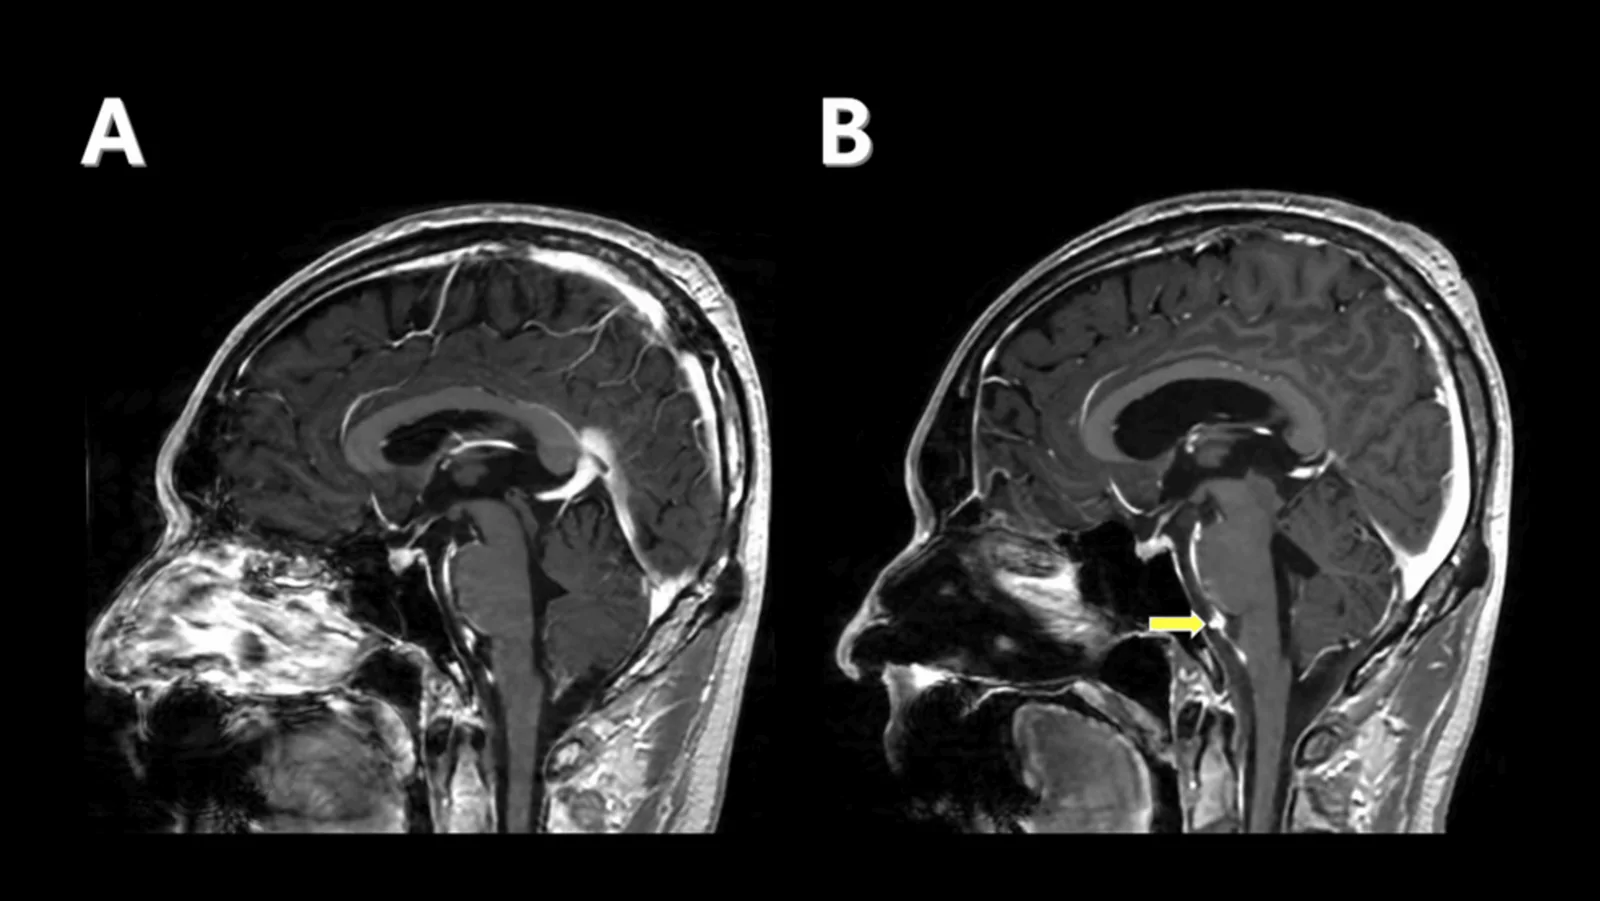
Comparison of follow-up contrast-enhanced brain MRI scans (A) A contrast-enhanced brain MRI from the year before the onset of SAH shows no outpouching lesion near the VB junction. (B) A follow-up MRI performed in the year of the SAH event reveals a suspected aneurysm near the VB junction (yellow arrow). The appearance of this lesion closely coincides with the timing of the rapid surge in beta-hCG levels MRI: magnetic resonance imaging; SAH: subarachnoid hemorrhage; VB: vertebrobasilar; hCG: human chorionic gonadotropin
